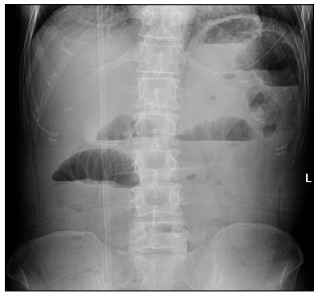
Abdominal radiograph: multiple gas-liquid planes in the intestinal gut.
